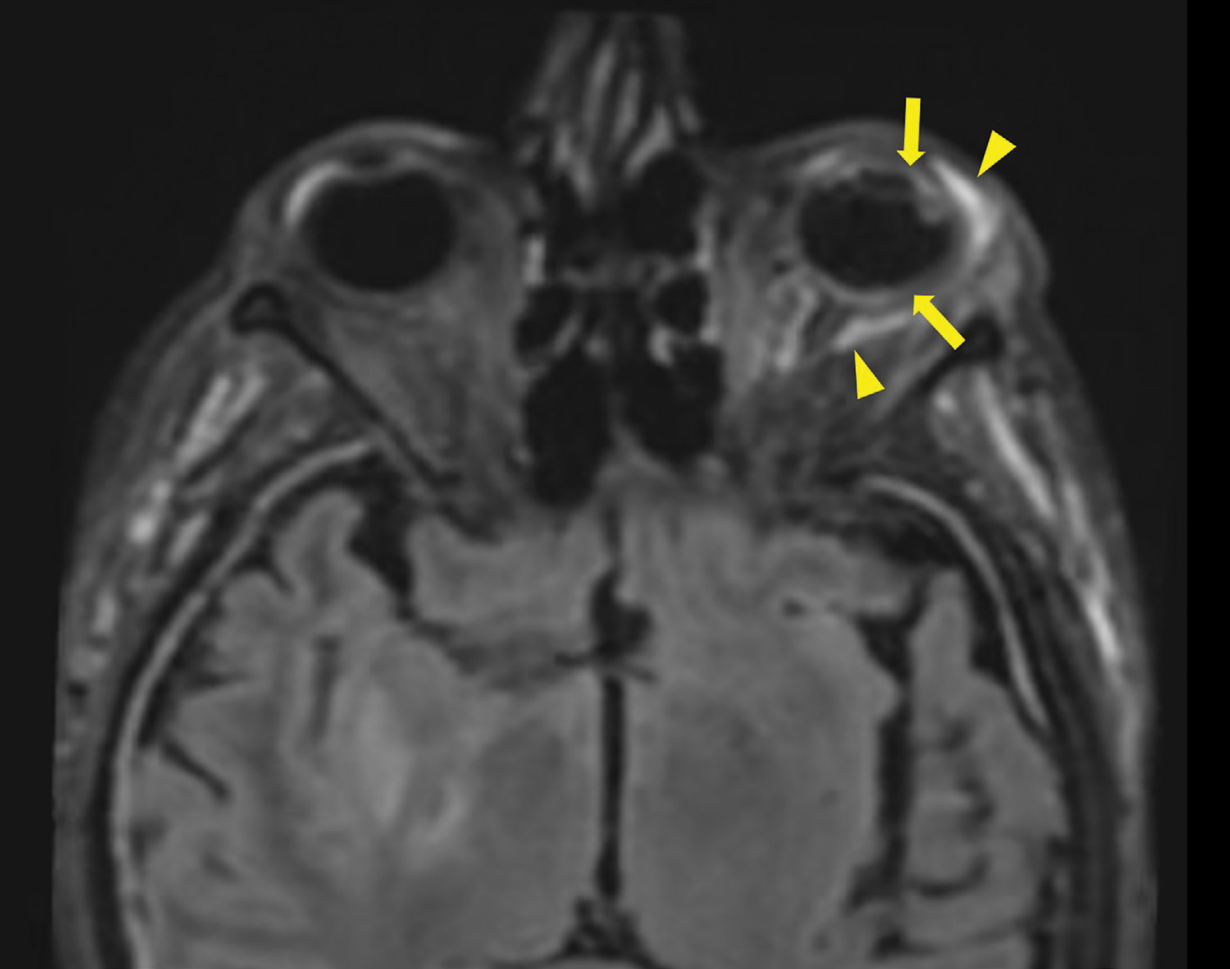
3D FLAIR Sequence with Fat saturation post contrast injection showing a pre‑septal and a perioptic hypersignal (arrowheads), a increase signal of the choroid and of the ciliary body. Also note the thickened dura mater in relation to the meningitis.
